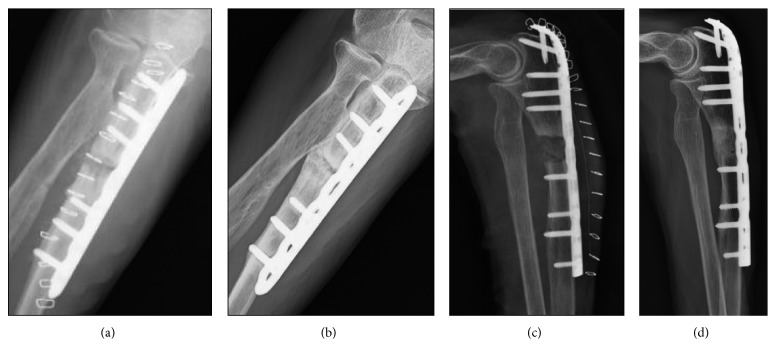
Plain radiographs of case 1 taken immediately after surgery (a) and 1 year after surgery (b) and case 2 taken immediately after surgery (c) and 1.5 years after surgery when bone union was observed gradually (d).
